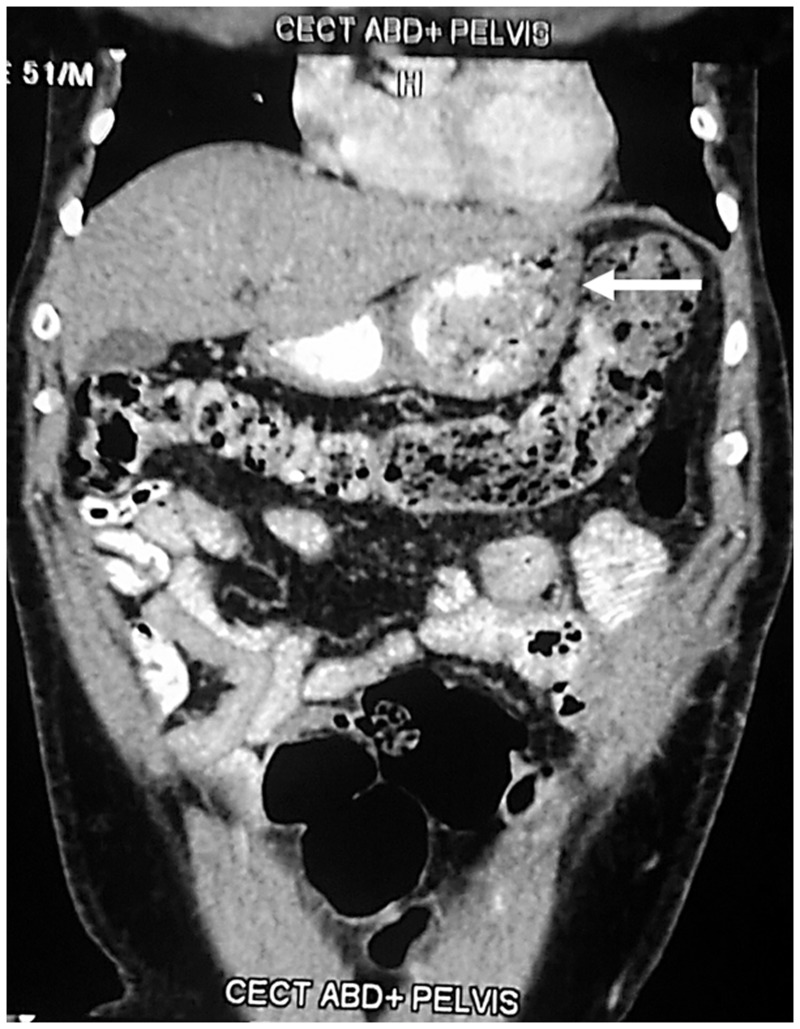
Abdomen and pelvic CT scan at 6 months follow-up reveals no residual disease or recurrence.
